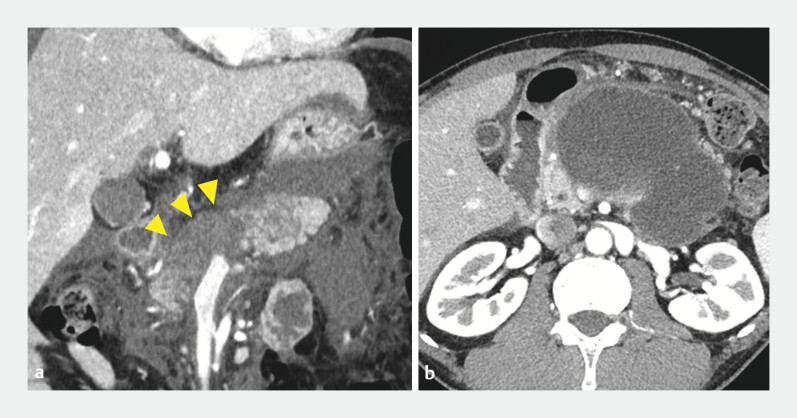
Contrast-enhanced computed tomographic images:
